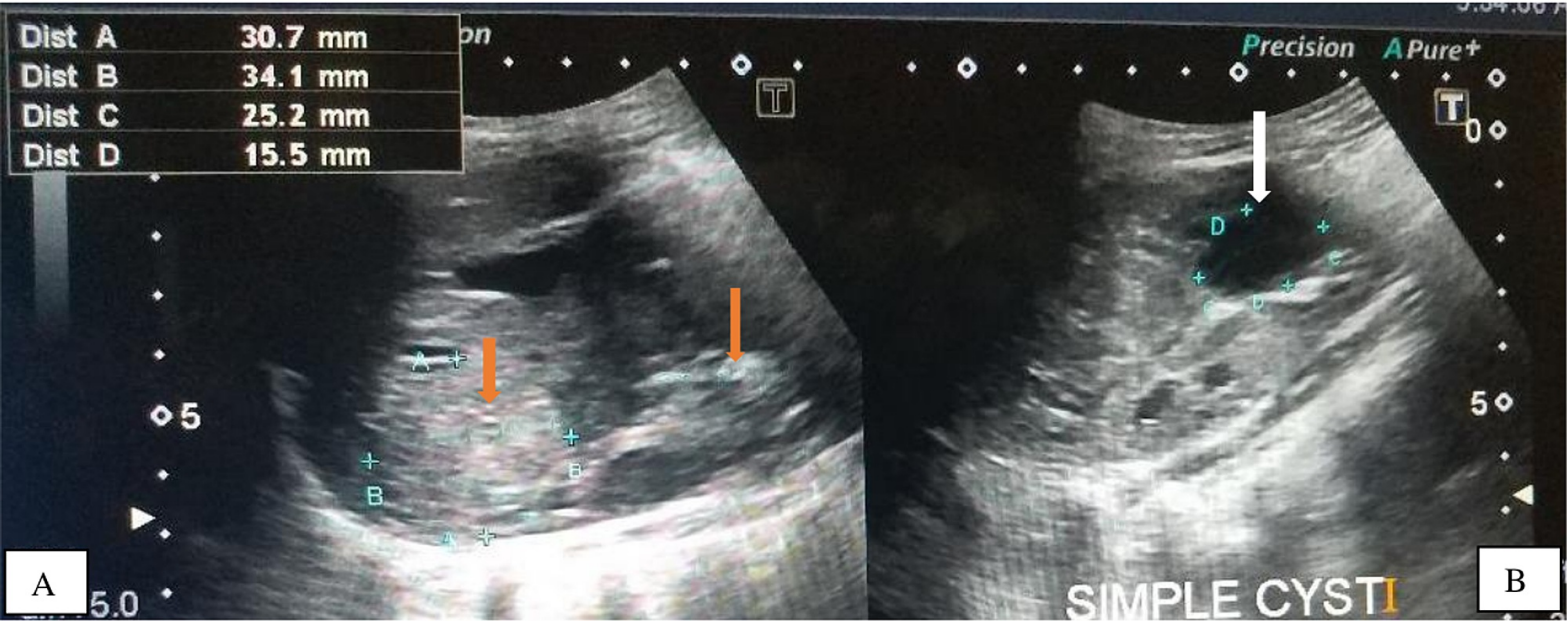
Ultrasound of the left Kidney showing the echogenic masses /angiomyolipoma (orange arrows) (9A) and the simple renal cyst (white arrow) (9B) respectively.
